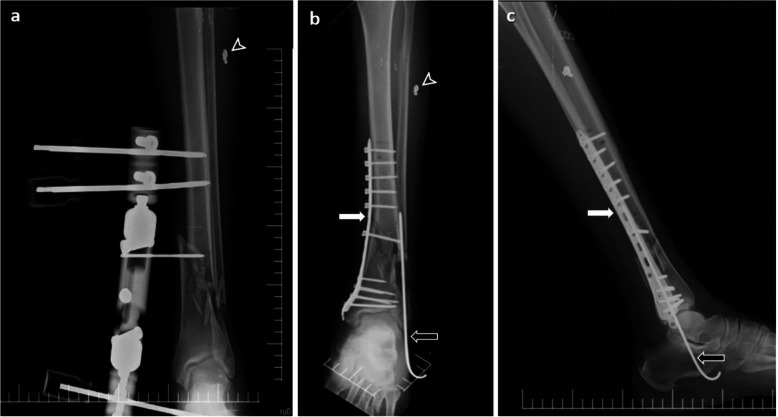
Images of a patient with type 3C open fractures in the tibia and fibula. Radiography image showing a the external fixator applied for bone stabilization and b-c Plate and screw placed in the tibia (white arrows) and intramedullary wire placed in the fibula (black arrows) for definite treatment. Penetrating fragments (arrowheads) are also present in soft tissue
